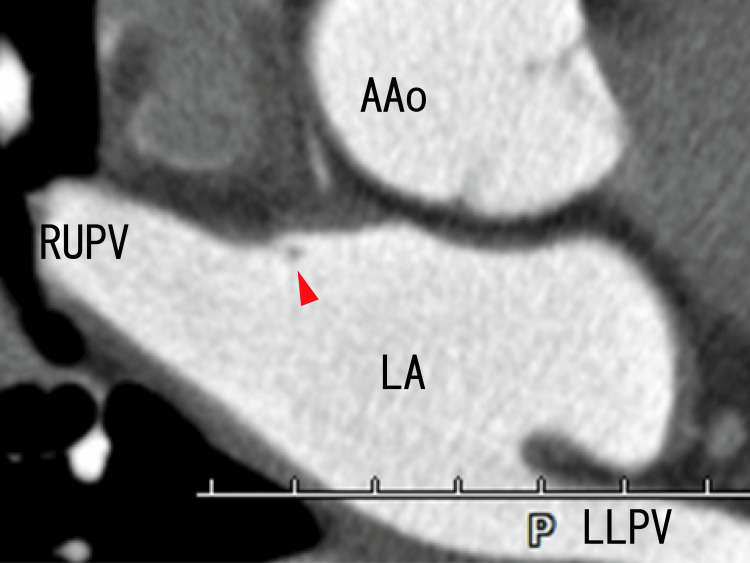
Axial images from an 80-MDCT scan revealing the attachment point in the LA Axial images from an 80-MDCT scan reveale the RUPV, LLPV, and LA. There were no images of thrombi in the LA. There was a dark spot near the anterior wall of the LA (arrowhead), suggesting that both the LA thrombi and the anterior wall of the LA were attached there. The dark spot and the anterior wall of the LA seemed to be connected by a dim thin line. 80-MDCT, 80-slice multidetector computed tomography; AAo, ascending aorta; LA, left atrium; LLPV, left lower pulmonary vein; RUPV, right upper pulmonary vein.
